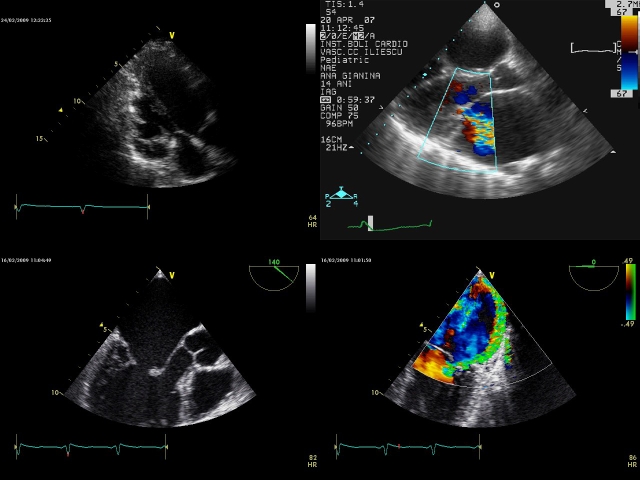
Two (2D) Transthoracic echocardiography:
